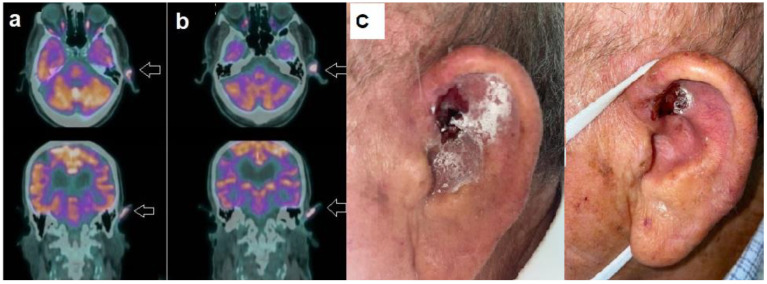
Patient n. 3, an 89-year-old-male, affected by advanced BCC. (a) Baseline PET/CT showed increased tracer uptake in the left ear (arrow), as well evident in the axial (upper row) and coronal (lower row) image. (b) Follow-up PET/CT after 8 months of HPI therapy, demonstrating stable metabolic disease according to PERCIST; (c) Clinical examination at the time of PET-1 (left side) and of PET-2 (right side), consistent with substantially stable disease.
